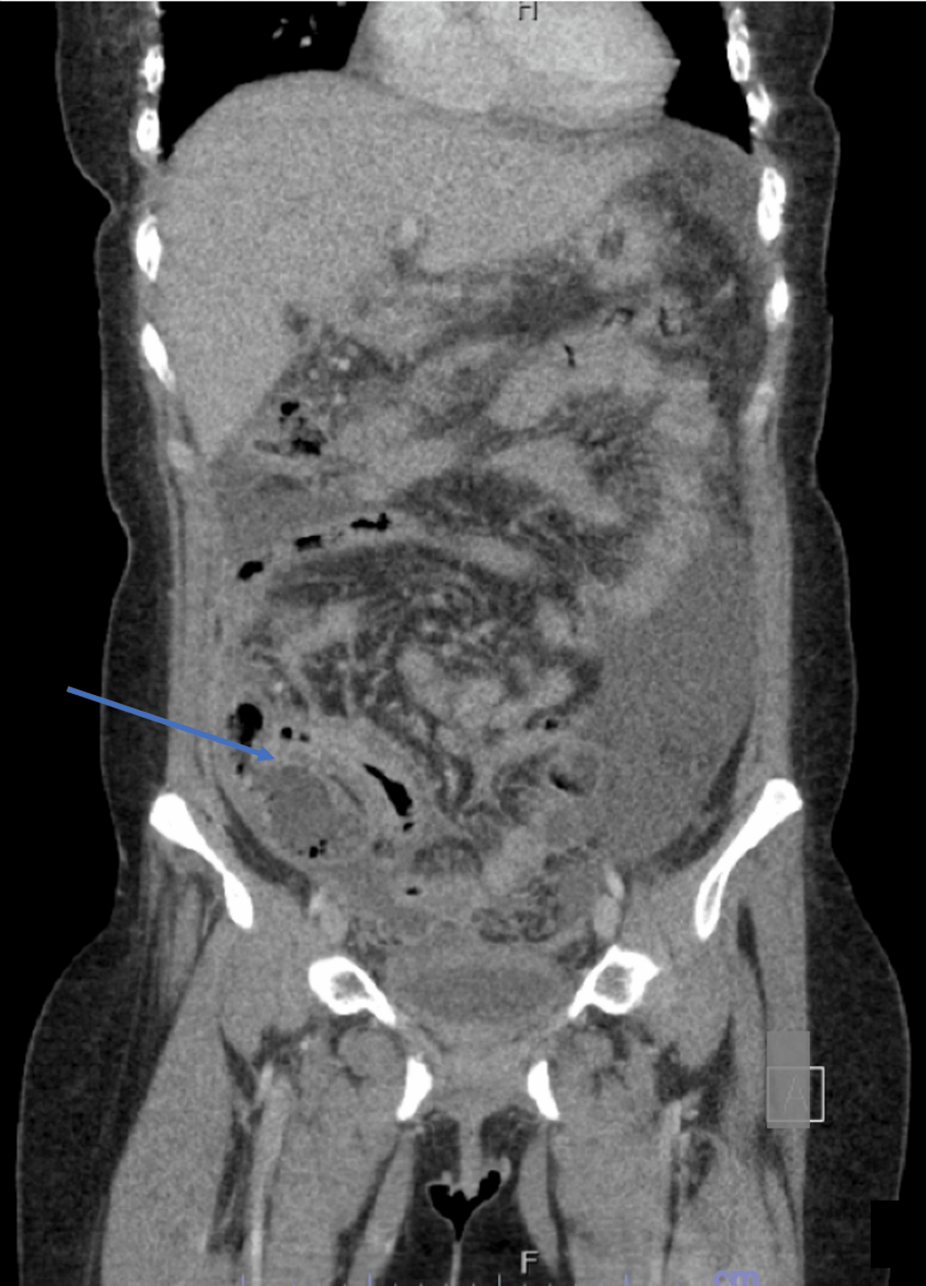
CT coronal images showing diffuse mural thickening and enhancement affecting the small bowel, cecum, and ascending colon CT: computed tomography
